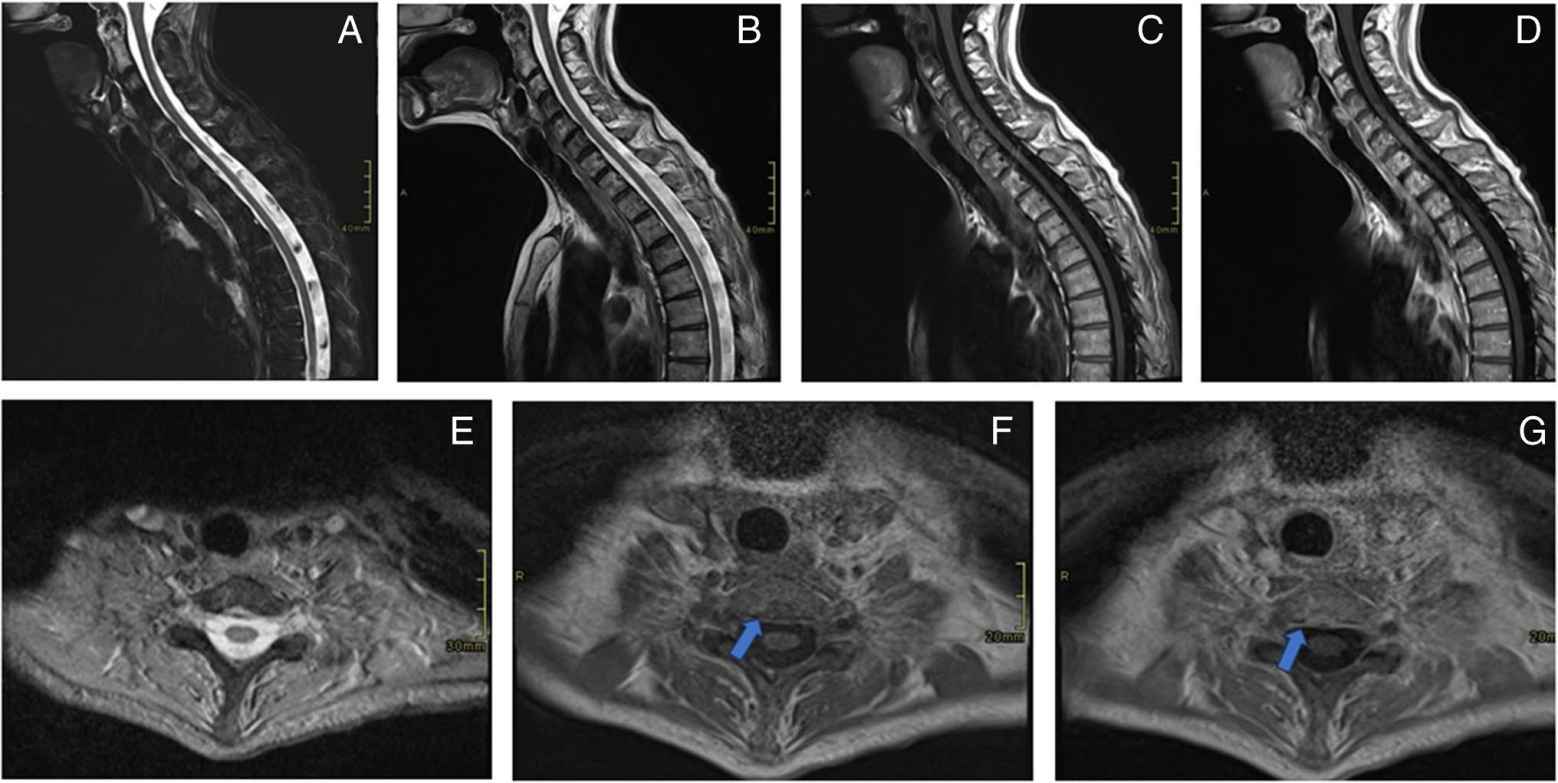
Sagittal midline short tau inversion recovery (STIR) image (A), T2 image (B), T1 precontrast (C) and postcontrast (D) images, and axial T2-weighted image at the T1 level (E) show mild nonspecific vertebral body marrow signal heterogeneity. Axial T1 precontrast (F) and postcontrast (G) images show T1 hypointensity and enhancement (arrows) in the region corresponding to the enhancement seen on previous neck CT images (see Fig. 1)
